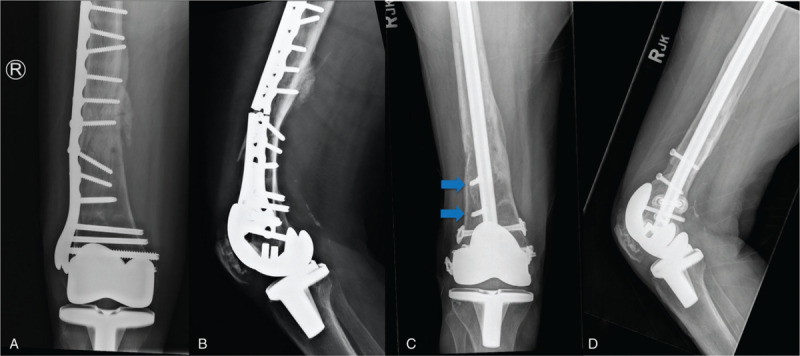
Periprosthetic distal femoral nonunion. Radiographs of 68-year-old female 9 months after open reduction and internal fixation of a periprosthetic distal femur fracture, demonstrating nonunion and plate breakage (A and B). The initial construct was overly rigid with too short of a plate, insufficient working length, excessive screw fill, and inadequate spacing of fixation. Postoperative radiographs 6 months after revision fixation using a retrograde intramedullary nail and bone morphogenetic protein (BMP, Infuse, Medtronic) (C and D). Note the use of blocking screws to restore anatomic alignment (blue arrows).
